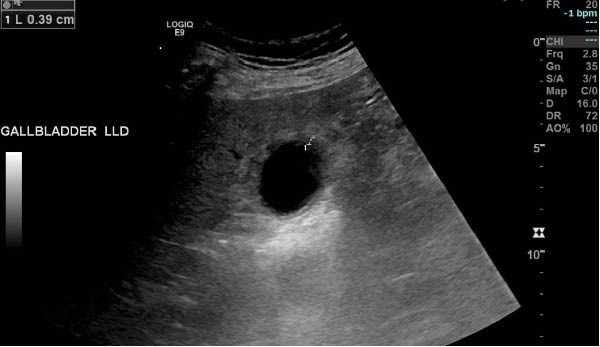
Ultrasound showing gallbladder wall thickness (0.39 cm).
